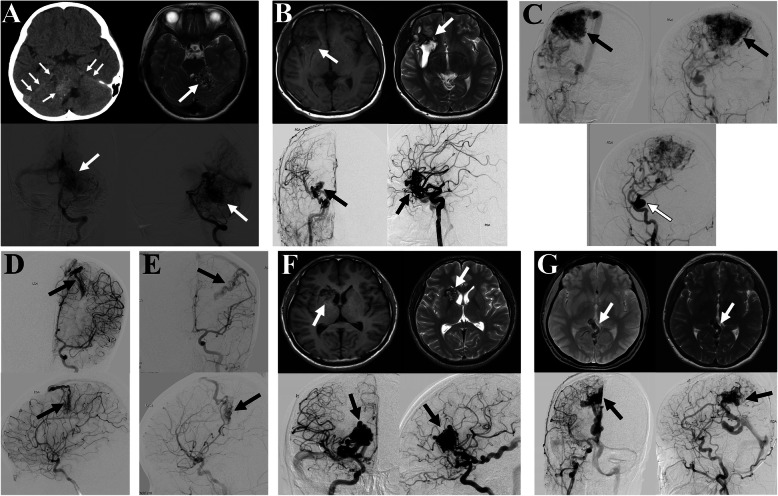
MRI, CT, and DSA imaging of patients carrying variants in syndromic genes. A Patient BAVM1 with *ENG* gene mutation c.901G>C. CT demonstrated subarachnoid hemorrhage in the distribution of posterior circulation, and DSA showed an AVM in the area of right cerebellar hemisphere. B Patient BAVM2 with ACVRL1 gene mutation c.1103C>T. MRI showed a hematoma and DSA showed an AVM in the right temporal lobe. C Patient BAVM3 with BMPR2 gene mutation c.775C>T. DSA revealed an AVM in the right side of the parietal lobe (black arrow) and an intracranial aneurysm in the internal carotid artery (white arrow). D, E Mutations of *BMPR2* c.2678G>A shared by BAVM4 and BAVM5. DSA showed an AVM in the left parietal lobe in two patients. F, G Patient BAVM6 and BAVM7with *RASA1* gene mutations c.1280G>A and c.3007G>A, respectively. MRI and DSA showed an AVM in the right basal ganglia and right parietal cerebral falx on two patients
